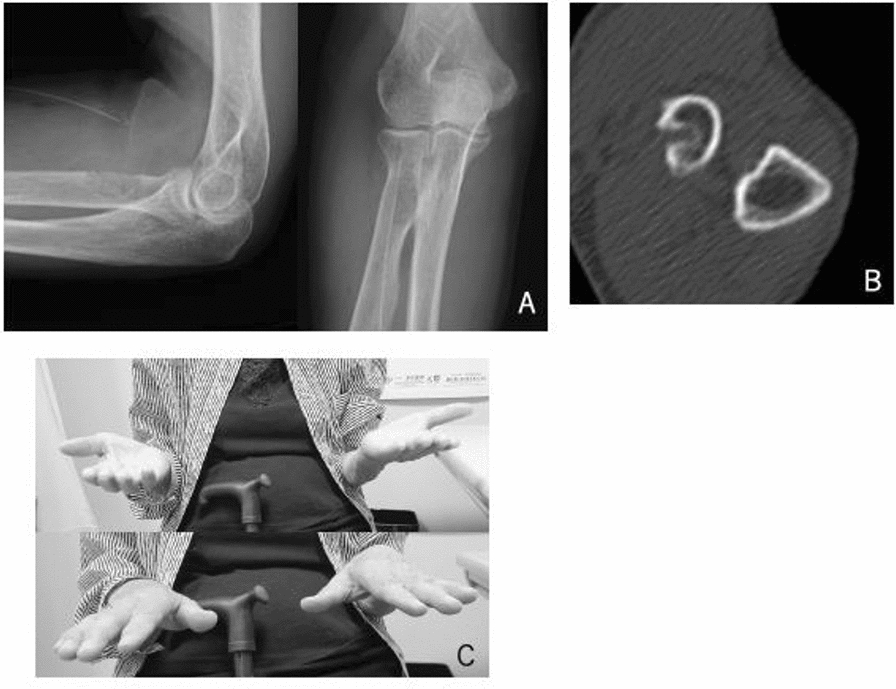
A Postoperative radiographs of the left elbow showed no abnormal prominence of the bicipital tuberosity of the radius. B Postoperative computed tomography of the left elbow showed no abnormal spur of the bicipital tuberosity of the radius. C The patient’s limitation of the forearm rotation of the left elbow improved
